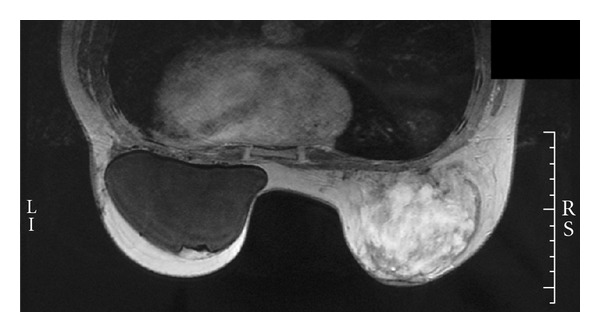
Magnetic resonance imagery showing the left breast implant and the highly vascular mass encompassing most of the right breast.
